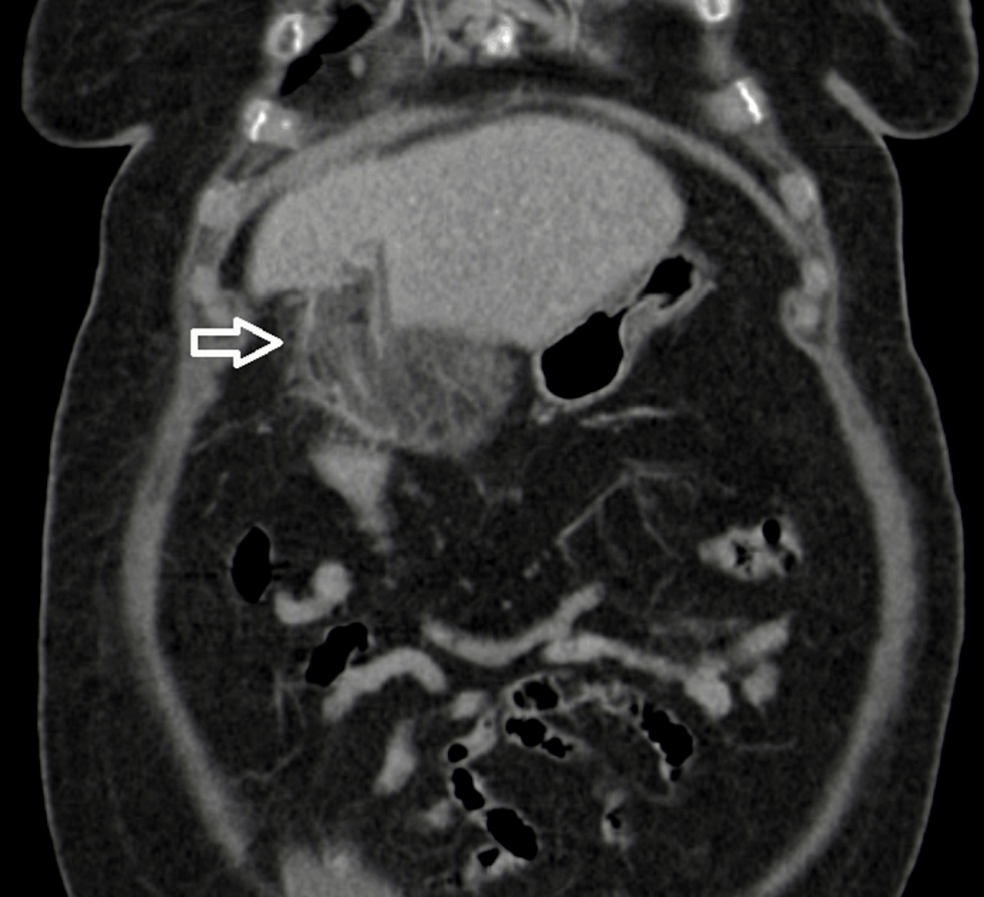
Coronal computed tomography reconstruction demonstrating the infarcted appendage (white arrow).
